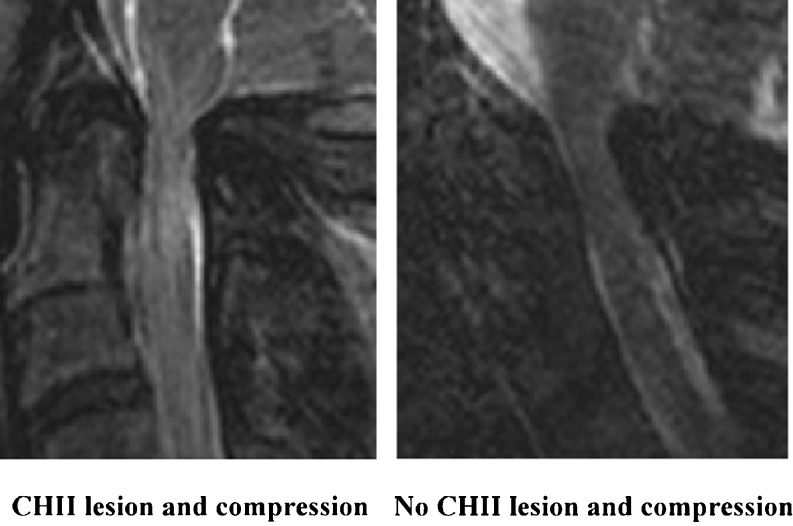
Compression of the spinal cord at the craniocervical junction. MR images of the two subjects who exhibited compression on the spinal cord at the craniocervical junction in the neutral position
